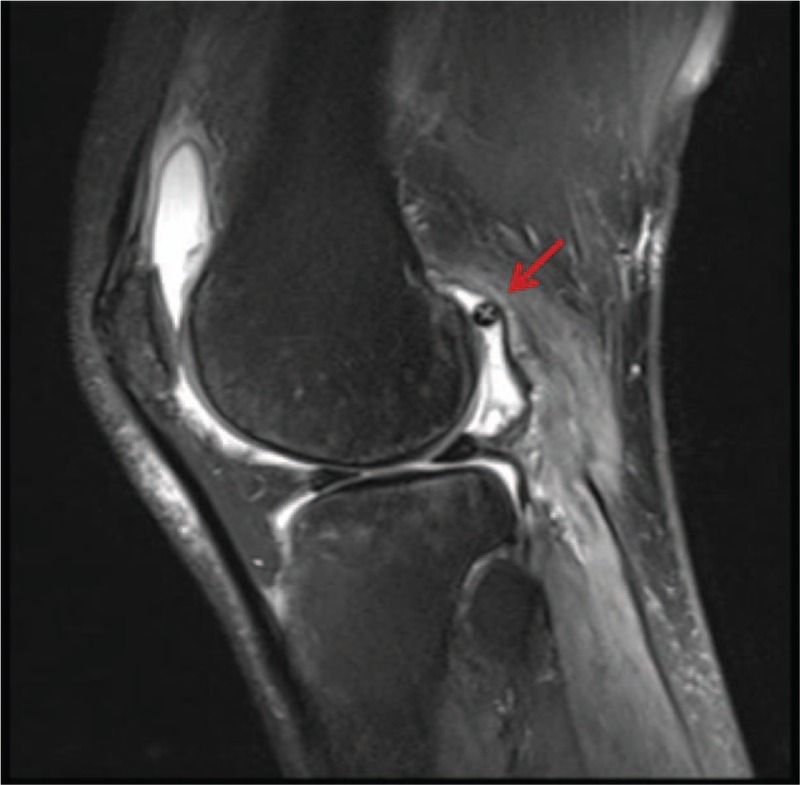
MRI 5 months after the initial operation revealing osteochondral lesions on the tibial and femoral sides and the head of the interference screw lying in the back of the joint capsule compartment (red arrows).
